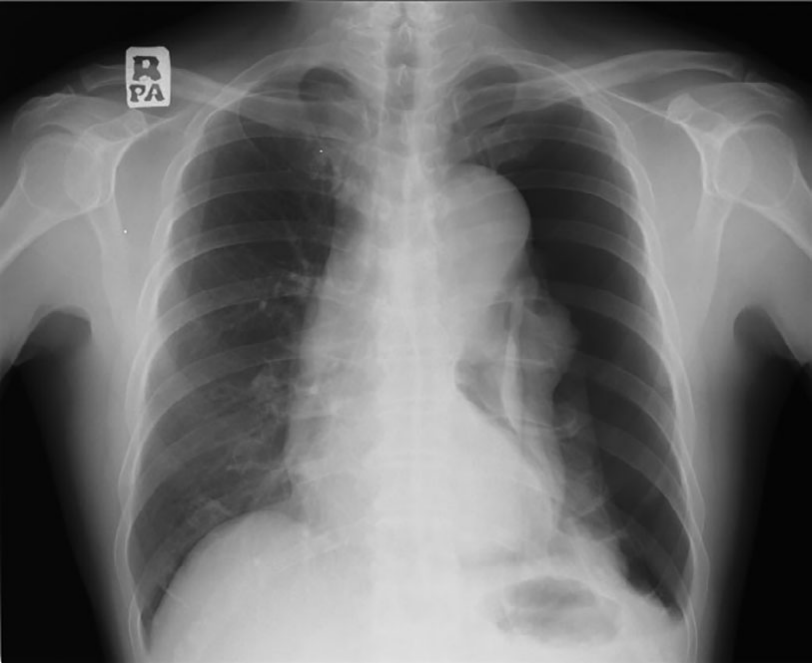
Chest radiograph showed left pneumothorax without trachea deviation.
